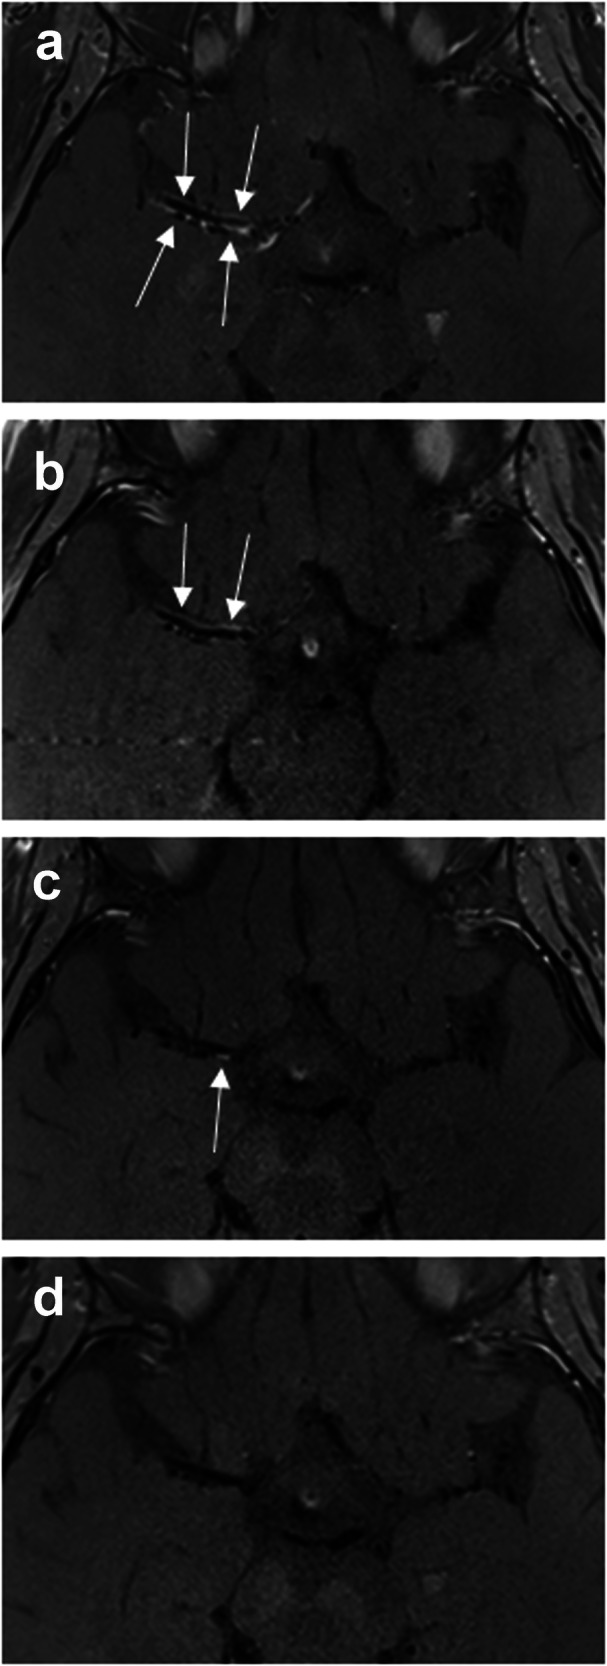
T1-weighted dark blood turbo spin echo MRI with intravenous contrast agent in a 29-year-old female patient with primary angiitis of the central nervous system of the right middle cerebral artery. Vessel wall enhancement (white arrow) decreases over time and finally disappears completely. a 13 days before immunosuppression, (b) 4 days after treatment start, (c) 235 days after treatment start, (d) 377 days after treatment start. Note the complete disappearance of the vessel wall enhancement over a long time
